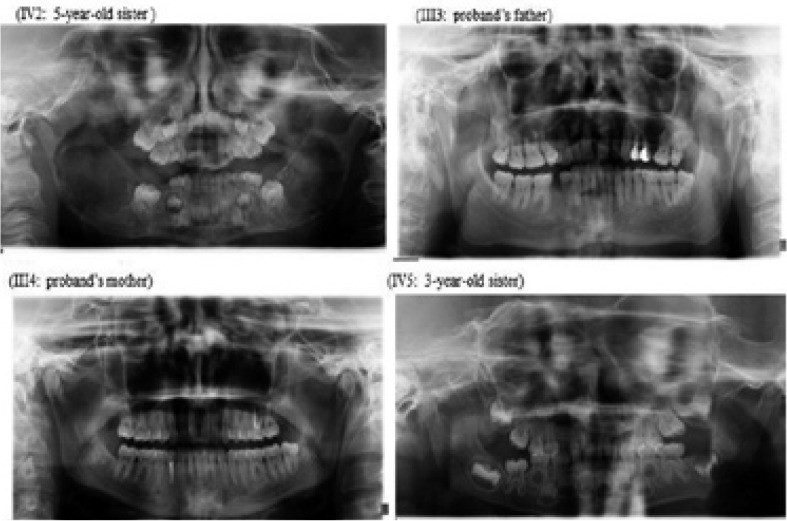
Panoramic radiographs of proband’s family members. Cherubism patient IV2 and clinically and radiographically normal little sister (IV5) and parents III3 and III4.
